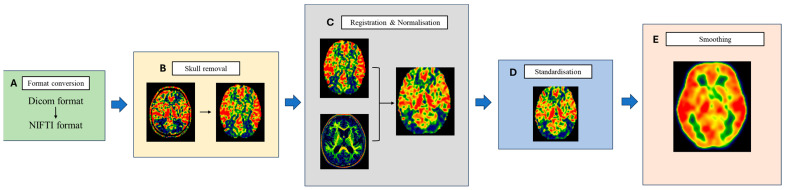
ASL-MRI processing streamline. (A) Image format conversion: all the imaging of patients are converted from Dicom format to NIFTI format. (B) Skull removal: removing the extracerebral parts of the CBF images. (C) Registering the CBF map onto the T1 images and normalising them to the Montreal Neurological Institute (MNI) template. (D) Standardisation of CBF values of the whole brain with the mean division method. (E) Spatially smoothing of CBF maps: spatial-filtering techniques are applied to smooth the feature map to eliminate local noise while maintaining the global trend.
